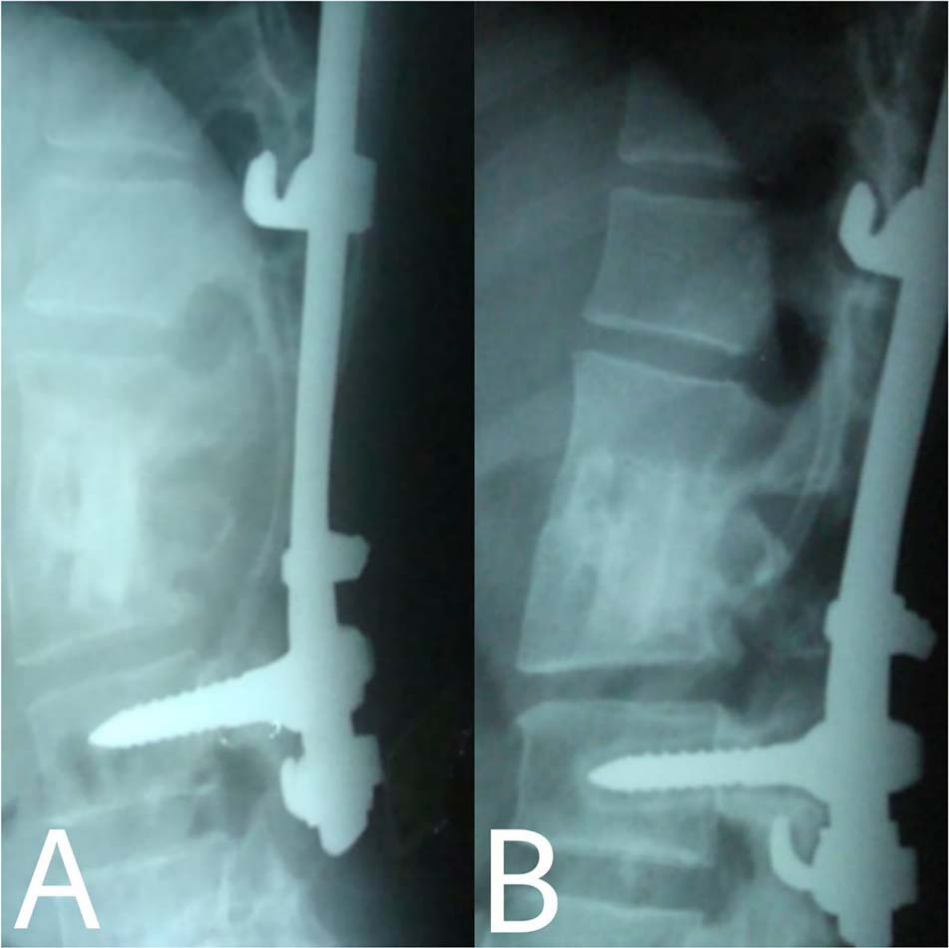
(A, B) Complete bony incorporation between the implanted fibular autograft and host vertebral body was noted on the lateral radiograph 1, and 14 years later.
